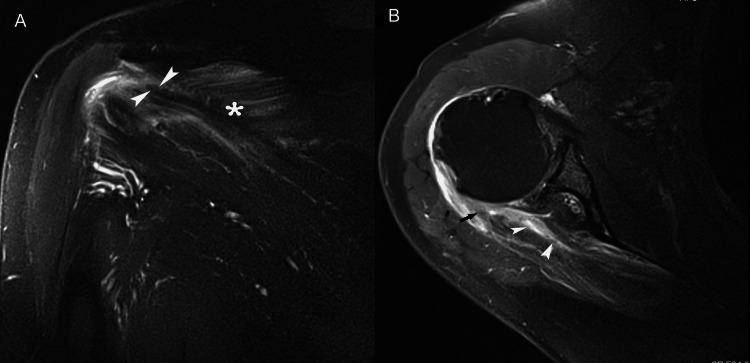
A 23-year-old man presented with pain in the right shoulder after an intense weight-lifting workout. (A) Coronal short-tau inversion recovery image shows abnormal high signal intensity in infraspinatus muscle, without obvious architectural distortion of muscle. Edema in a “feathery” pattern is tracking along muscle fascicles (asterisk) and around epimysium (arrowheads) between cranial and central parts of the infraspinatus. (B) Axial fat-suppressed fast spin-echo T2-weighted MR image reveals hyperintense fluid within the muscle belly (arrowheads), consistent with a strain of the infraspinatus muscle. Note extensive edema at the myotendinous junction (arrow) of the torn infraspinatus tendon.
